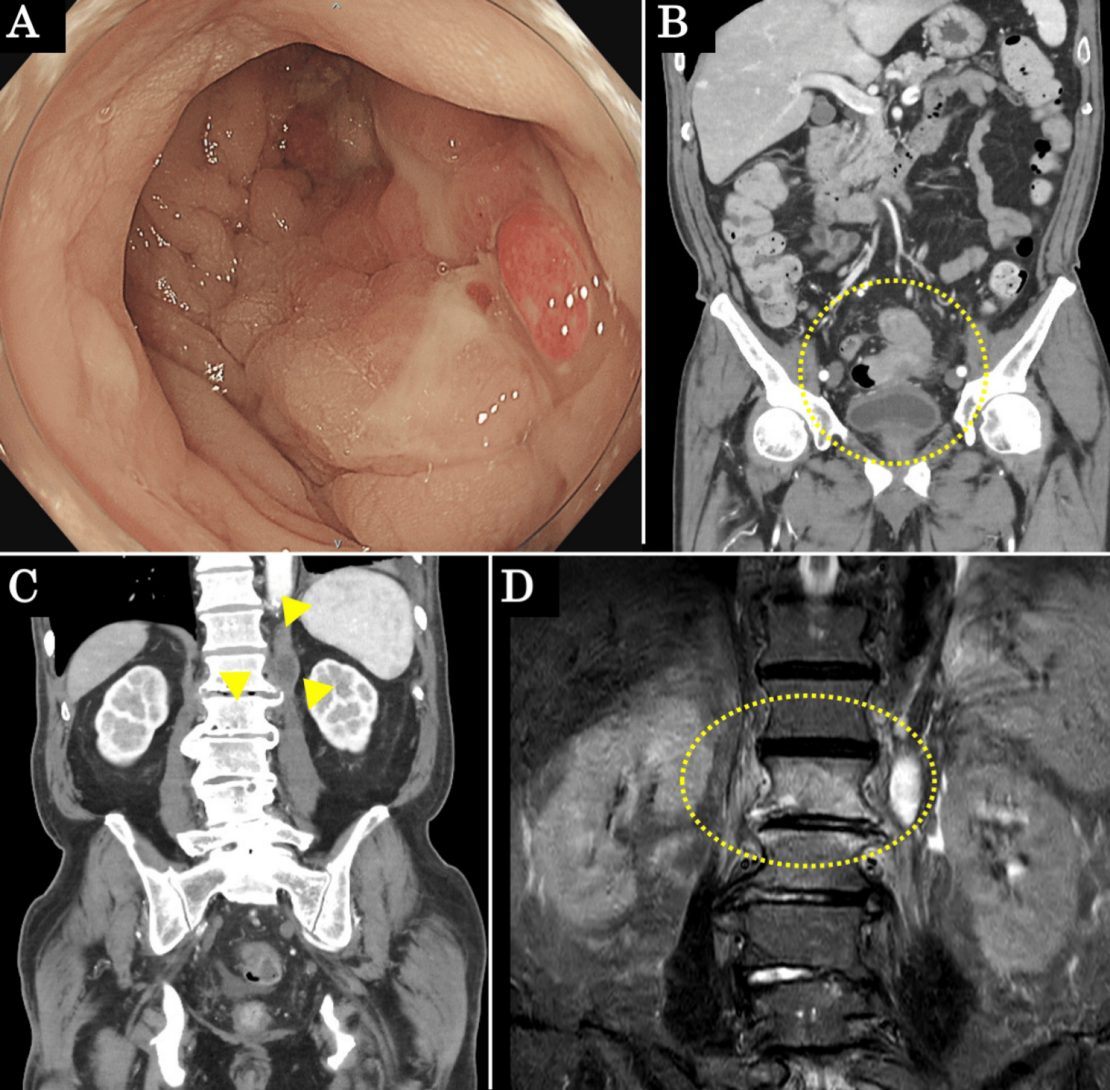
Preoperative endoscopy, contrast-enhanced CT, and MRI findings (A) Endoscopic findings revealed a circumferential tumor in the rectosigmoid. (B, C) Contrast-enhanced CT revealed wall thickening in the rectosigmoid, which was in contact with the small intestine and bladder (yellow dotted area). Fluid collection was observed on the left side of the L1 vertebral body (yellow arrows). (D) T2-weighted MRI revealed a high-intensity area on the L1 and L2 vertebral bodies, as well as the left side of the L1 vertebral body. (yellow dotted area).
